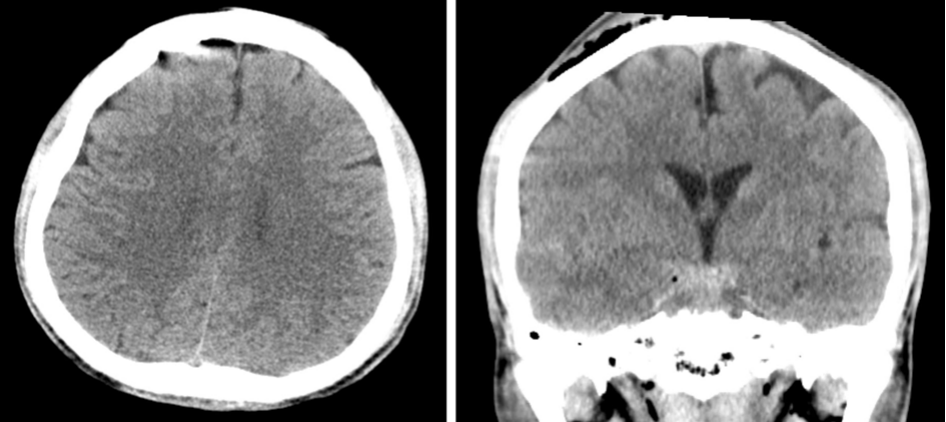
Initial CT of the head. Left, axial view; right, coronal view shows subarachnoid hemorrhage. Lack of midline shift is noted. CT, computed tomography.
